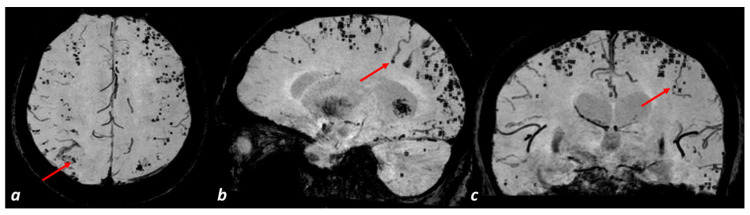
Multiple cortical MBs in a patient with CAA in SWI-MRI reconstructed in axial (a) sagittal (b) and coronal (c) plane. The red arrows point to the potential connection of MBs with cortical venules.
